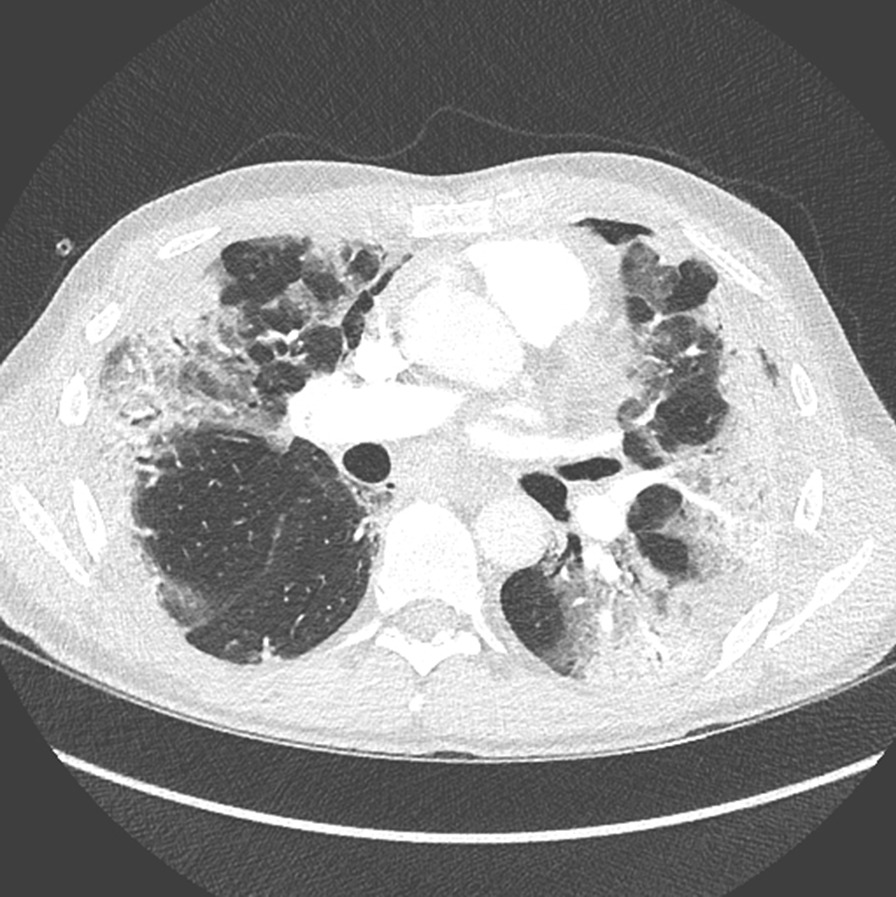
CT scan after 2 weeks of hospitalisation. In comparison with the initial CT scan, ground glass opacities have been replaced by much more extensive consolidation lesions, especially predominant at the bases. Majoration of bilateral consolidations present in the middle and inferior lobes
